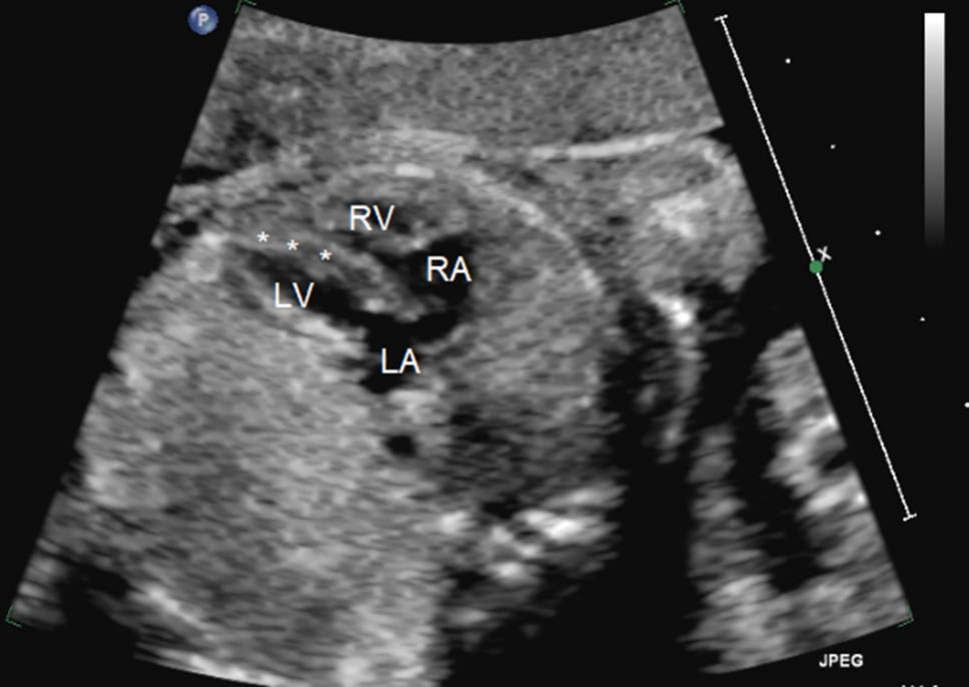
Fetal echocardiographic four-chamber view showing the abnormal horizontal position of the interventricular septum (*) as a result of the superior inferior rotation of the heart. *LV* left ventricle, *RV* right ventricle, *LA* left atrium, *RA* right atrium
